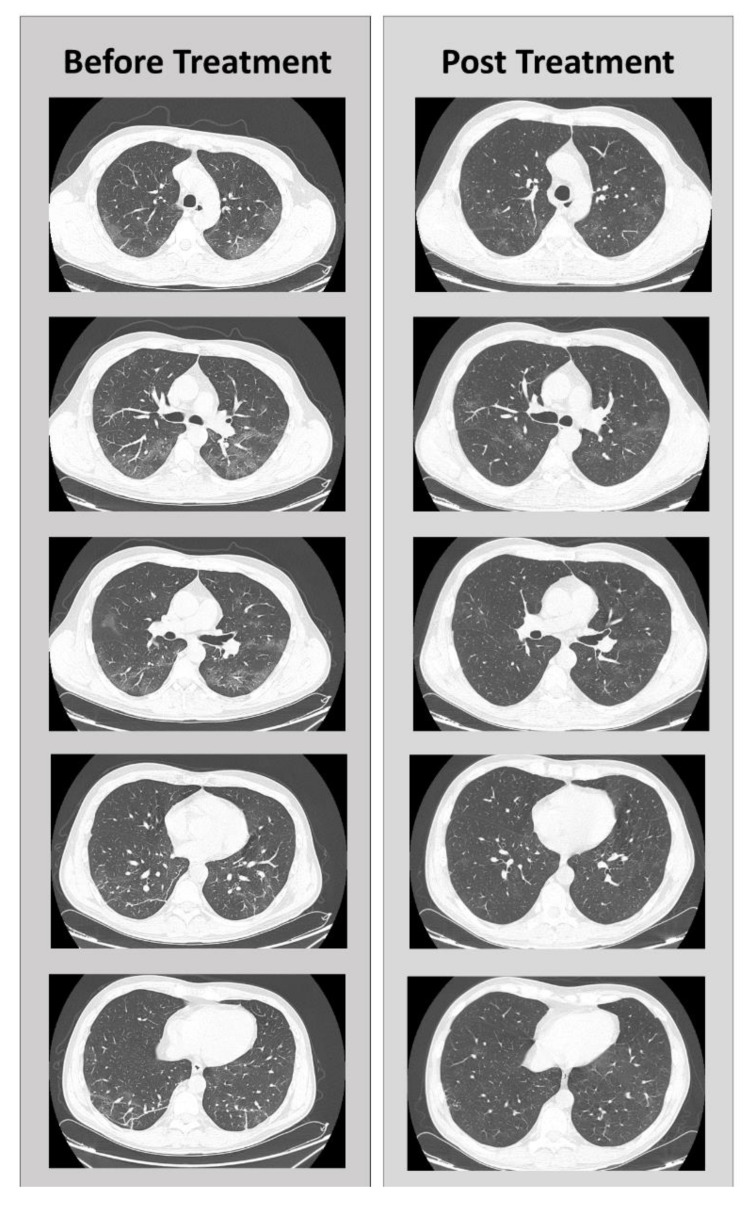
Comparison of CT scans before and after adenosine treatment, in different sections.
